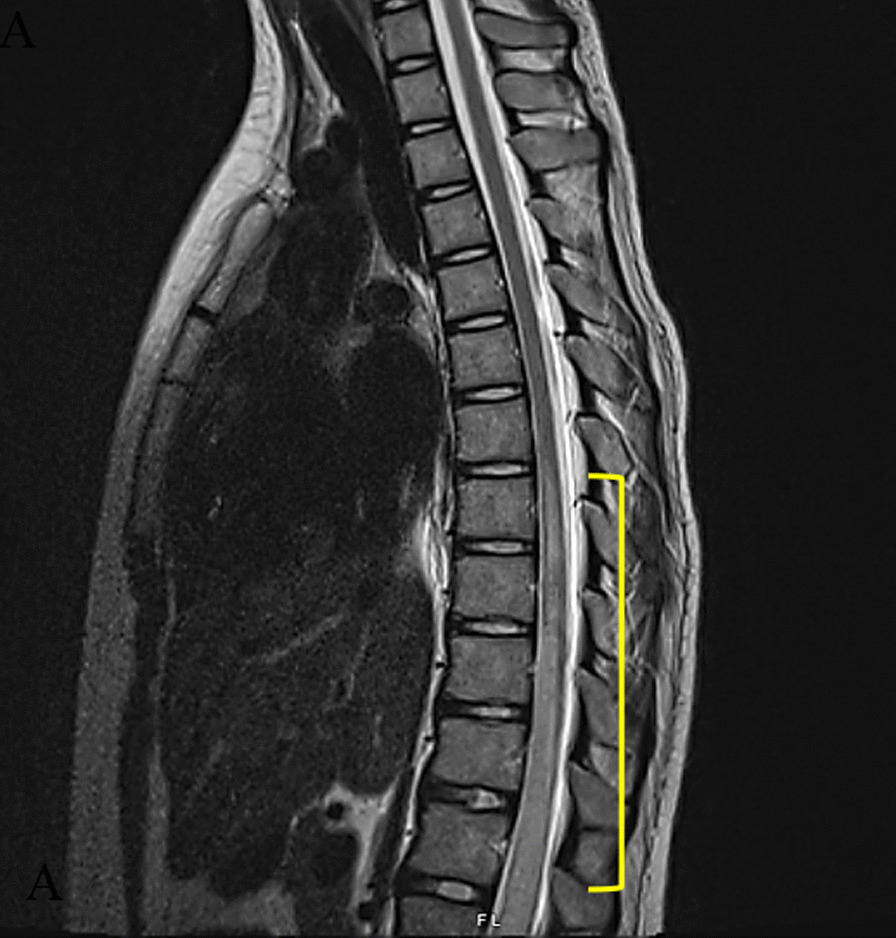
Image obtained at time of diagnosis of TM. T2-weighted sagittal MRI of thoracic spine demonstrating signal abnormality without abnormal enhancement extending from T7 to L1 with cord edema and expansion most prominent at T9–L1 (bracket)
